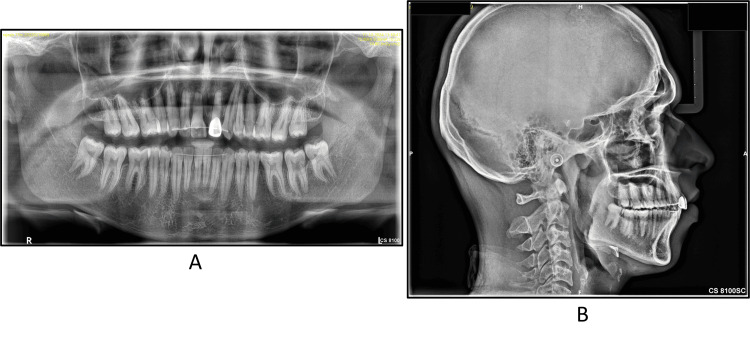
Posttreatment (A) OPG and (B) lateral cephalogram OPG: orthopantomogram
